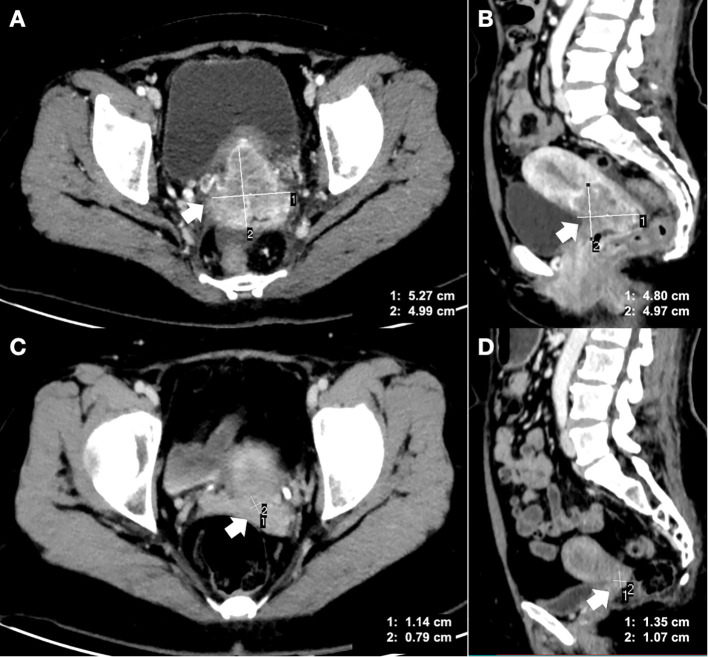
Comparison of enhanced CT images at pre-treatment (2025.09.04) and pre-surgery (2025.11.24). (A) Tumor mass in the cervix at the transverse position (5.27 cm×4.99 cm) prior to treatment. (B) Tumor mass in the cervix at the sagittal position (4.80 cm×4.97cm) prior to treatment. (C) Tumor mass in the cervix at the transverse position (1.14 cm×0.79 cm) prior to surgery. (D) Tumor mass in the cervix at the sagittal position (1.35 cm×1.07 cm) prior to surgery. In each subfigure, the white arrow points to the tumor mass. CT, computed tomography.
